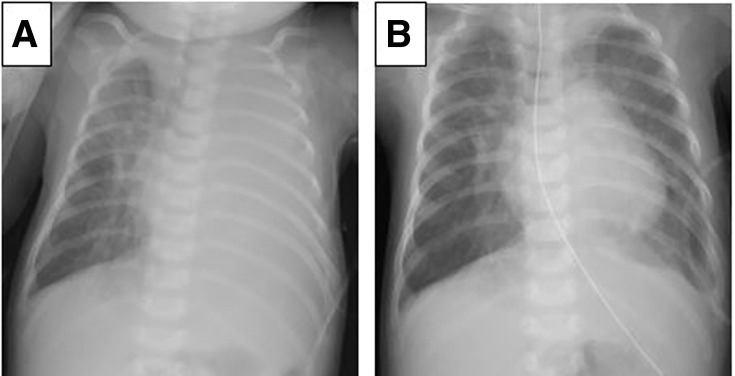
(A) Chest x-ray on post-operative day 16 showed unilateral pleural effusion. A diagnosis of chylothorax was made based on the biological examination of the pleural effusion. (B) The chest x-ray on discharge showed no pleural effusion on both sides.
